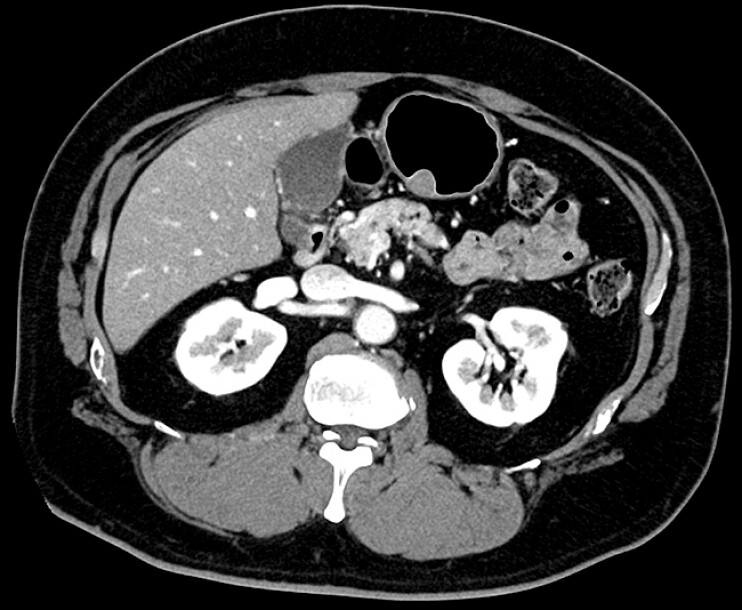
Computed tomography revealed a 25-mm gastric submucosal tumor and no evidence of metastasis.
